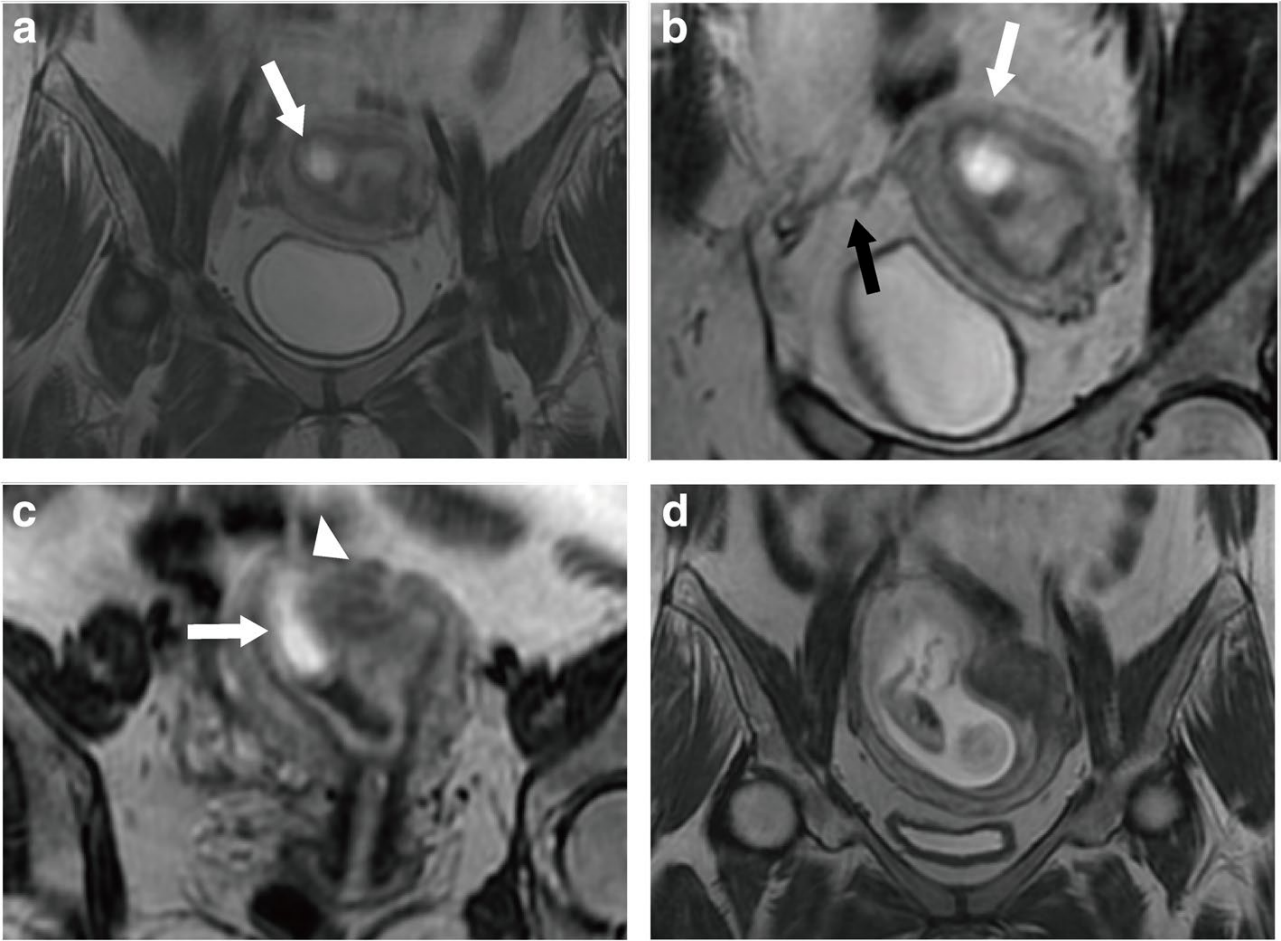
Right angular pregnancy in a 27-year-old woman at 7 weeks of gestation. The coronal 3D-CUBE T2WI images (a), reconstructed oblique coronal image (b) and reconstructed oblique axial image (c) revealed a cystic GS-like mass (white arrows) located at the right uterine angle and the mass was surrounded by T2 hyper/isointense endometrium. Figure b showed the GS was medial to the round ligament (black arrow). Figure c showed an interrupted junctional zone (white triangle) and the uterus cavity was asymmetric. An angular pregnancy was diagnosed according to these MRI features. The patient wanted to maintain pregnancy expectantly. After 6 weeks, this patient suffered a spontaneous abortion. The coronal Cube T2-WI(d) demonstrated the placenta was located on the right horn of the uterus and the fetus was indistinct. She underwent a C&D with ultrasound supervision
